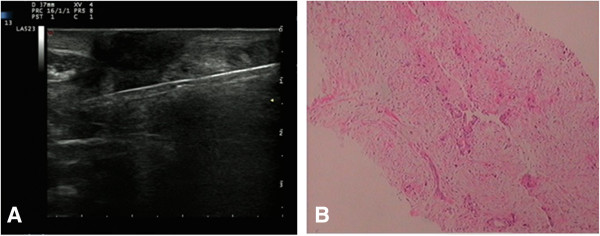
Core needle biopsy of the umbilical mass: a moderately differentiated adenocarcinoma. A: Ultrasound-guided core needle biopsy; B: Pathological diagnosis for the mass.
